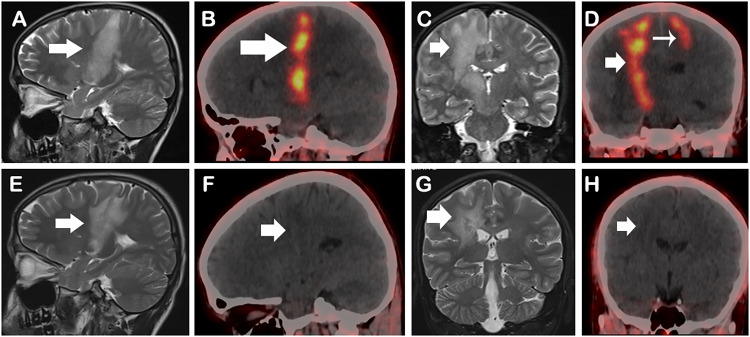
Corresponding MRI and ^68^Ga- FAPI PET/CT images in the same patient mentioned in Fig. 1, before (A-D) and after treatment (E-H). MRI T2/FLAIR sagittal and coronal images, demonstrated T2 hyperintensity along the corticospinal tract from the cortex to the subcortex, tracking along the white matter tract to the midbrain, a finding termed as “Tunnel Sign” (arrows in A, C). ^68^Ga FAPI PET/CT sagittal and coronal images show, tracer-avid (SUVmax 5.6) linear hyperdense areas involving the right frontal lobe, extending to the right basal ganglia and thalamus with significant perilesional edema (arrows in B, D). Another focal tracer uptake with a subtle hyperdense lesion in the left high frontal cortex, SUVmax 3.1, which is better appreciated on FAPI PET-CT (thin arrow in D). Follow-up MRI images post treatment completion show residual anatomical changes (arrows in E, G). However, there is complete resolution of activity on corresponding FAPI PET-CT images (arrows F, H). Patient improved significantly clinically post treatment
